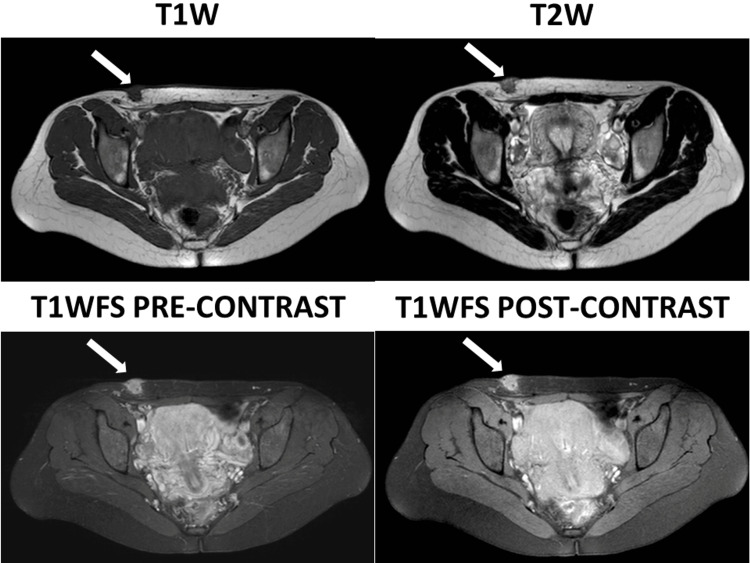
Magnetic resonance imaging of the pelvis showing an anterior abdominal wall lesion (white arrows), highly suggestive of endometrioma.
